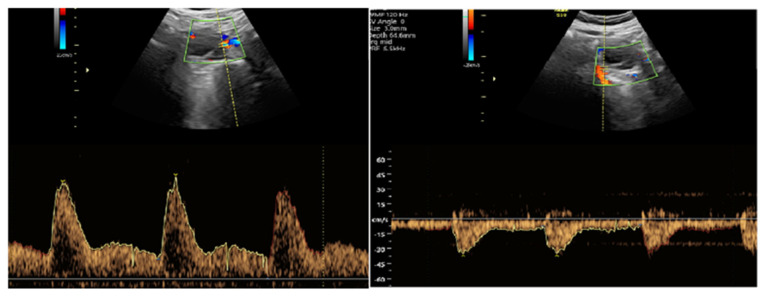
Corpus luteum power Doppler measurements.
